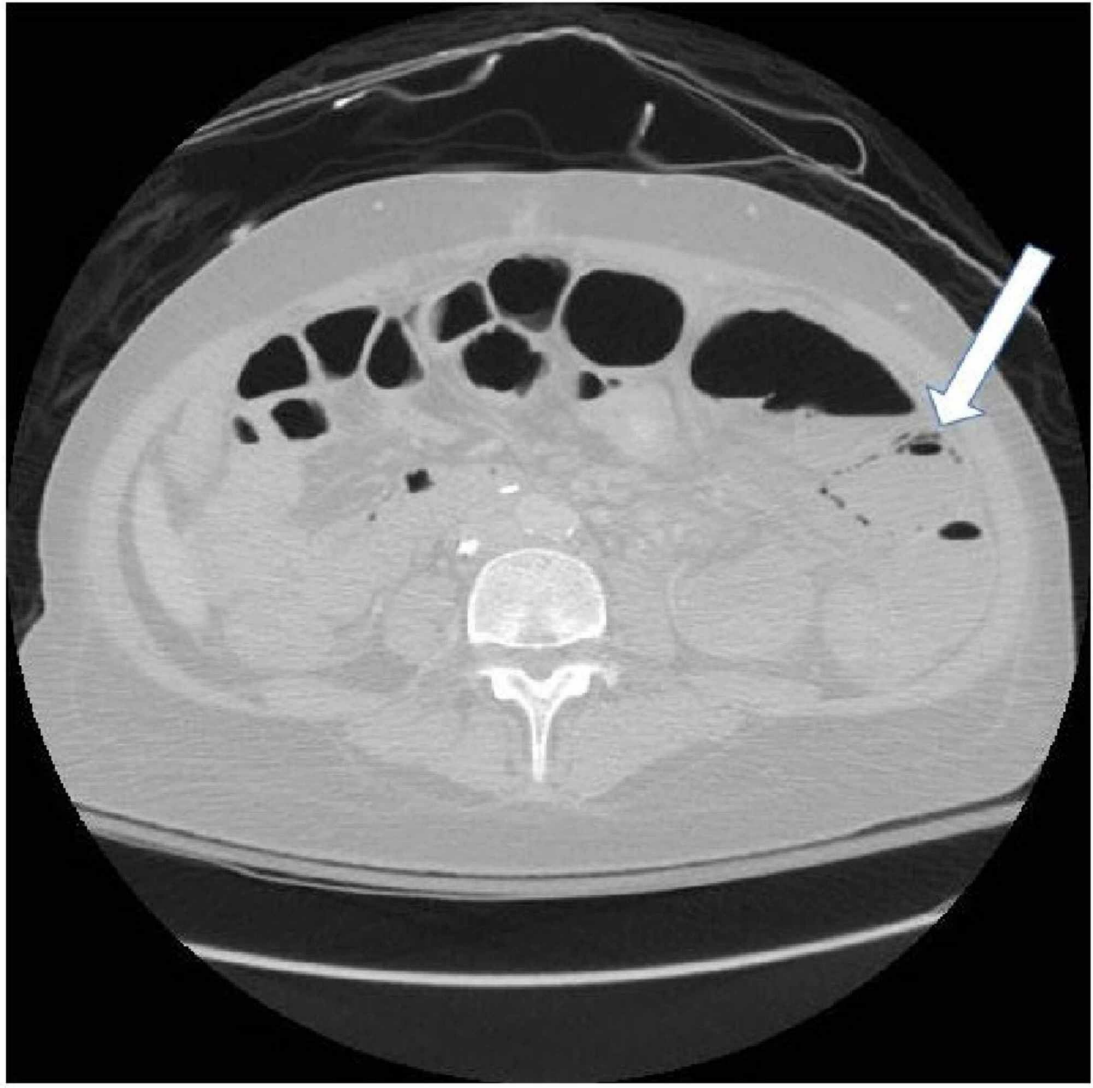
CT of the abdomen and pelvis with contrast findings showing pneumatosis involving a few borderline dilated loops of the small bowel in the lateral left mid-abdomen.
